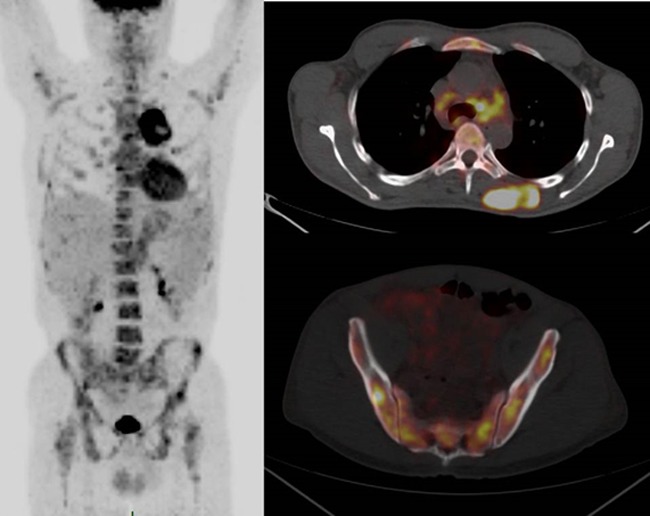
FDG-PET/CT in mast cell sarcoma The sites with confirmed disease are the bone marrow and axial and proximal appendicular skeleton (Figure 4). MIP (maximum intensity projection) image and axial fusion images. Soft tissue mass (dotted arrow) and mediastinal adenopathies (arrowhead) are shown here with multifocal bone marrow uptake.
